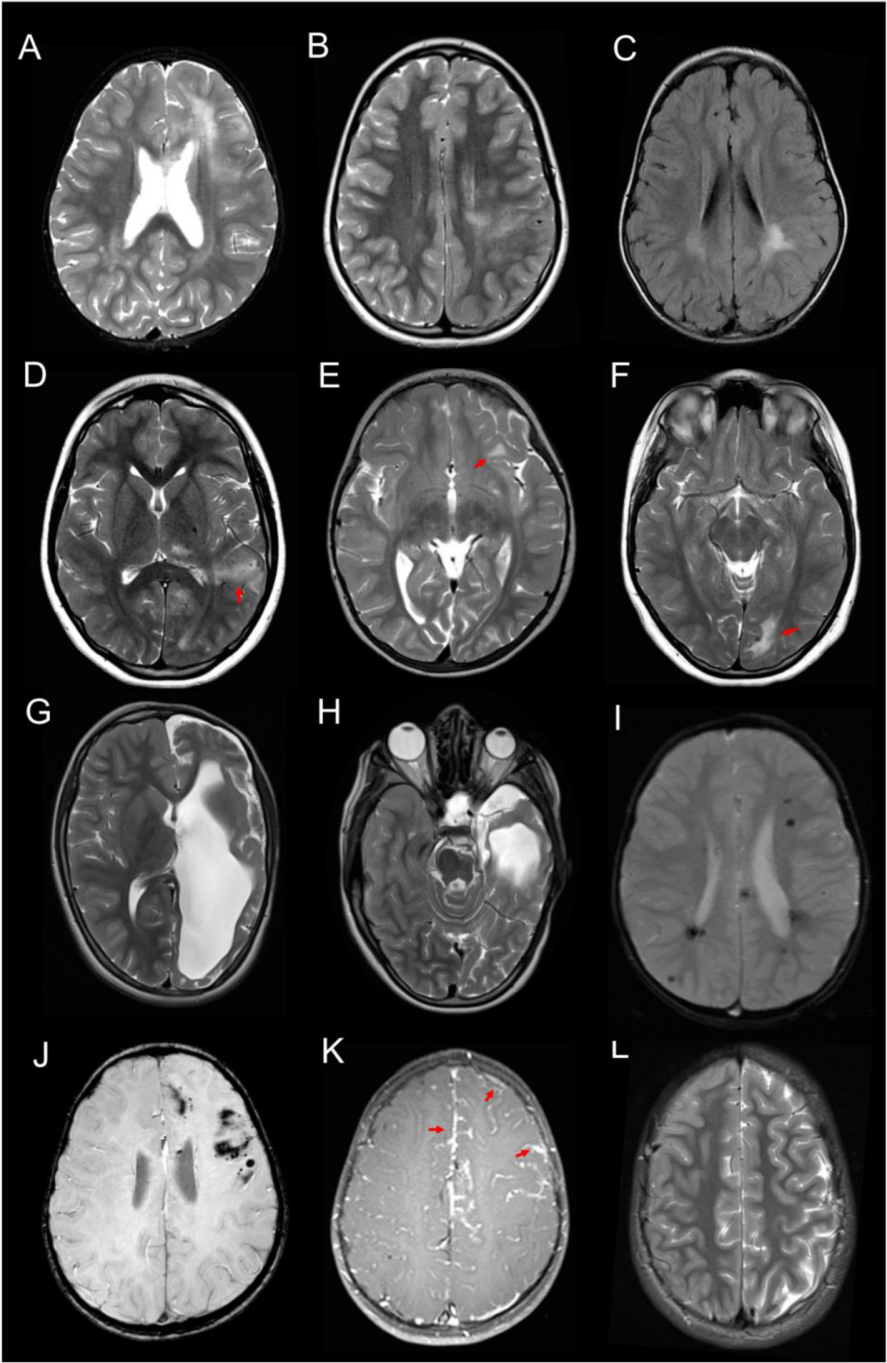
Magnetic resonance imaging (MRI) abnormalities in patients with Parry-Romberg syndrome and en coup de sabre. a-f T2-weighted and FLAIR MRI images showing enhanced white matter signalling. g-h T2-weighted MRI image showing porencephaly. i T2-weighted gradient echo MRI image showing multiple cavernomata. j T2-weighted gradient echo MRI image showing dystrophic calcification. k T1-weighted gadolinium enhanced MRI image showing leptomeningeal enhancement along the medial and lateral surfaces of the left frontal lobe. l T2-weighted MRI image showing sulcal crowding on the right
